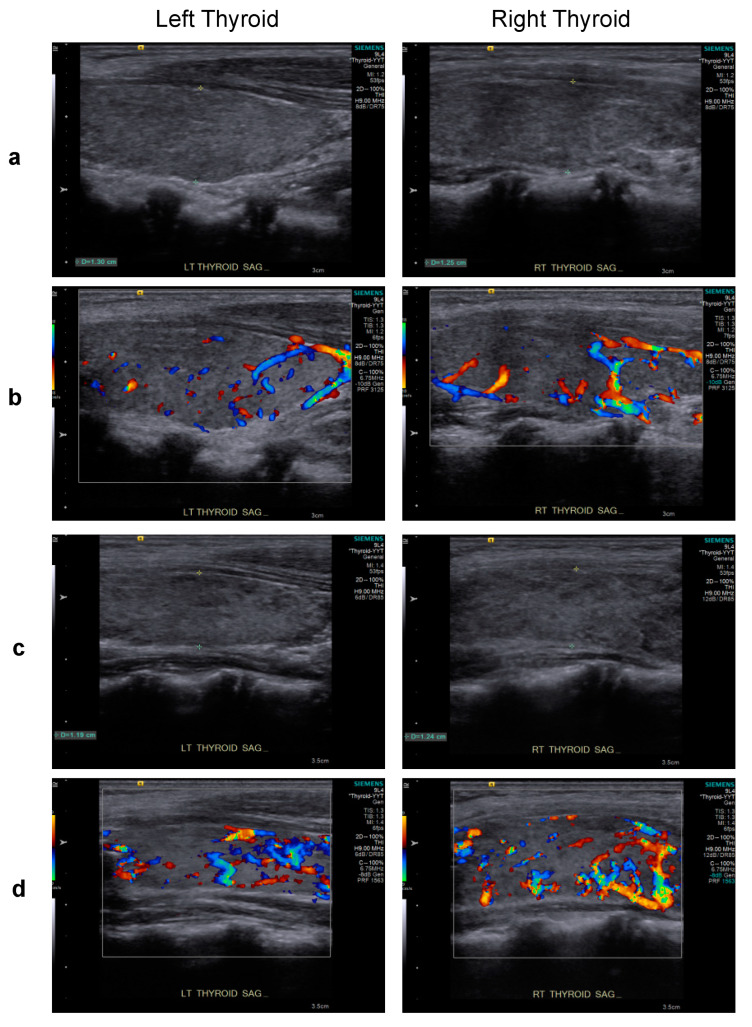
Upon presentation for hyperthyroidism, thyroid sonography of the patient revealed thyroid dimensions at the upper limit of the normal range and mildly heterogeneous parenchymal echotexture (grayscale sagittal images) (a) and hyperemia throughout the gland (color Doppler images) (b), features characteristic of Graves’ disease. A similar pattern of heterogeneous parenchymal echotexture (c) and hyperemia (d) were noted after 5 months of carbimazole treatment.
